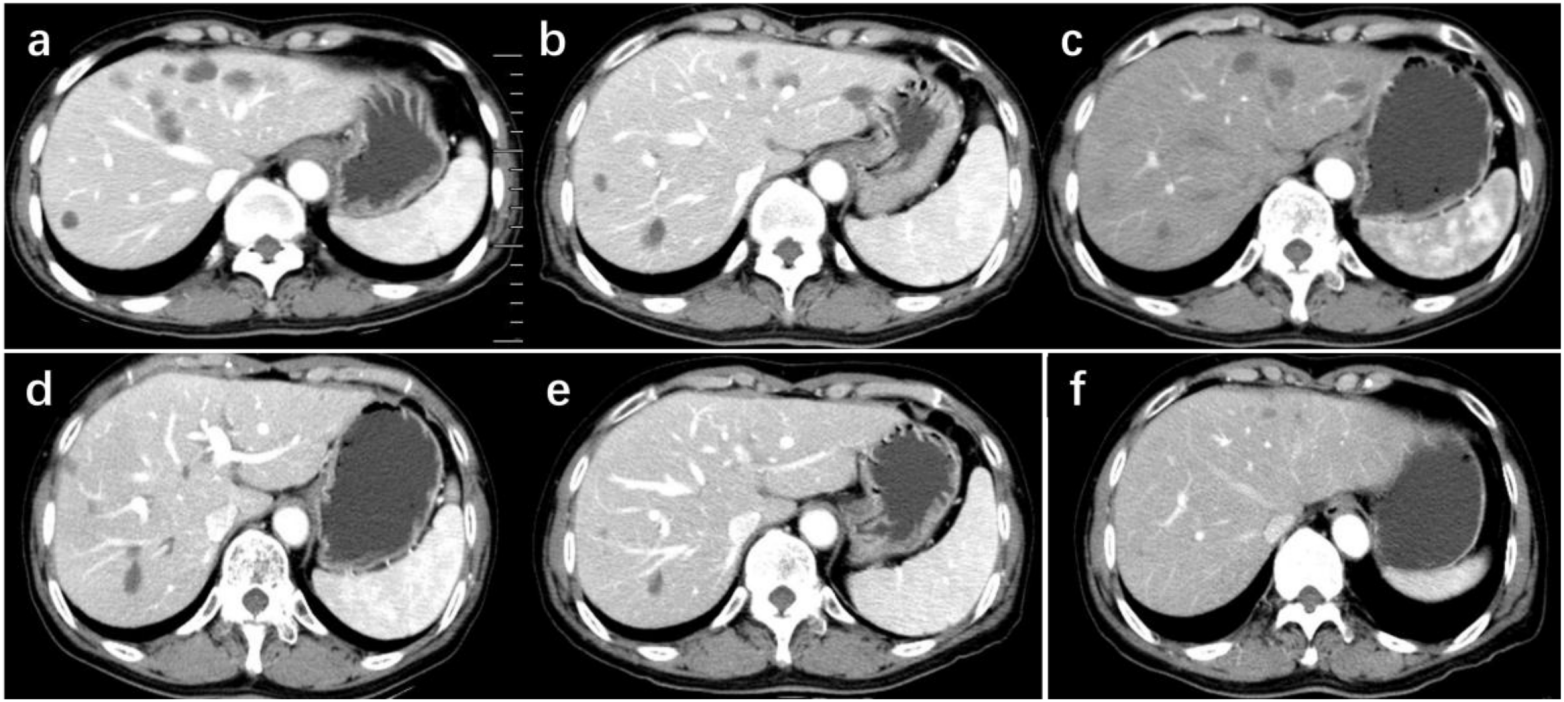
Serial contrast-enhanced CT scans demonstrated progressive reduction of hepatic metastatic lesions. The initial scans showed multiple billboard metastases on June 21, 2022 (a), November 2, 2022 (b), and January 5, 2023 (c). Follow-up imaging on March 6, 2024 (d), September 5, 2024 (e), and April 9, 2025 (f) revealed marked regression with minimal residual abnormalities and near-complete radiologic remission.
